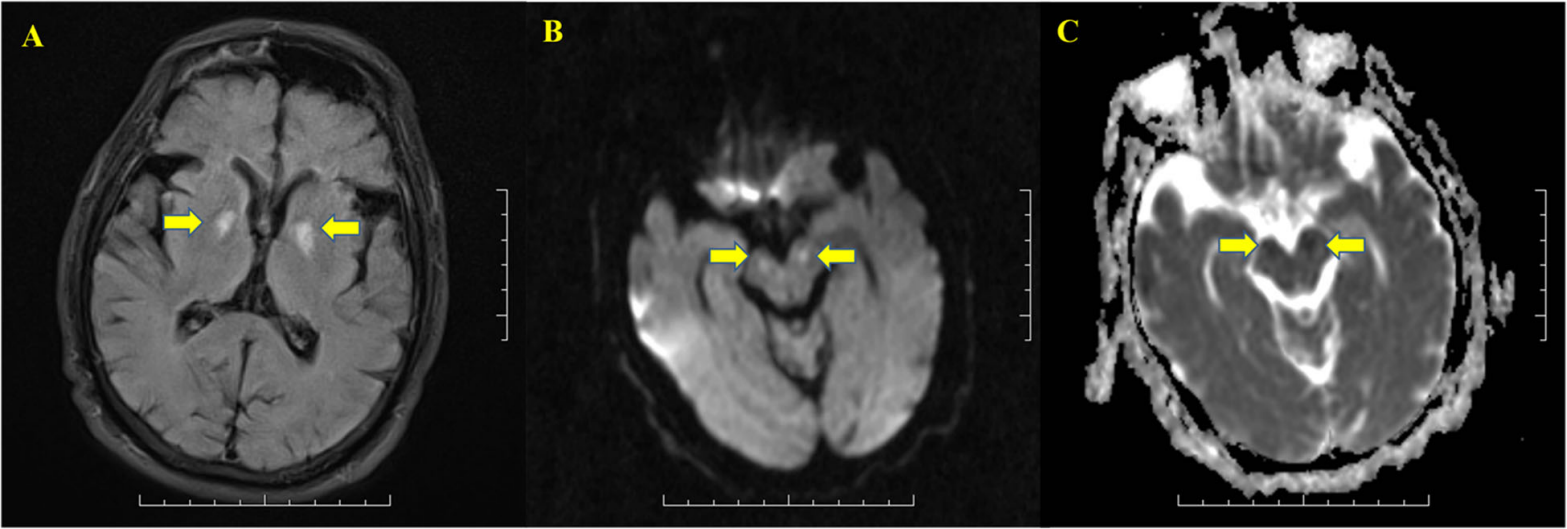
A bilateral symmetrical anomaly signal of the basal ganglia was captured in fluid attenuated inversion recovery images (a). Bilateral cerebral peduncles showed symmetrical high signals in DWI (b), but low signals in ADC
